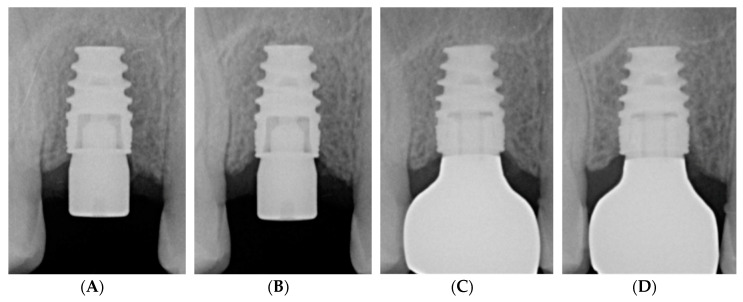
Radiographic follow-up of a NobelActive^®^ Wide Platform implant: (A) following implant placement; (B) after 3 months; (C) after 1 year; (D) after 3 years.
